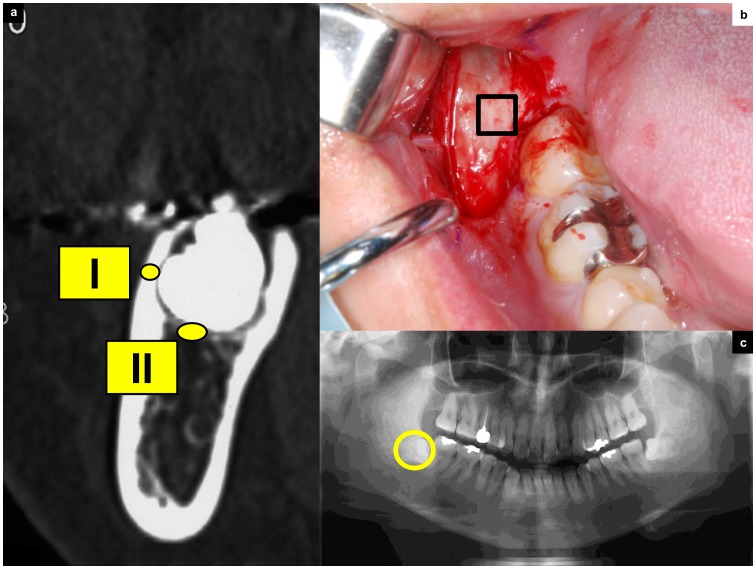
Design of the tooth extraction procedure and CT measurement. (a) This CT image details the condition of third molar teeth covered with cortical bone. The regions of interest in CT image analysis were (I) the buccal cortical bone area, which was taken for biopsy during surgery; and (II) the region under the cortical bone, which indicates cancellous bone near the third molar tooth. (b) Removal of the third molar tooth. The envelope flap is raised, revealing the cortical bone. The line indicates the biopsy area. (c) Orthopantomographs showing impacted third molar teeth.
